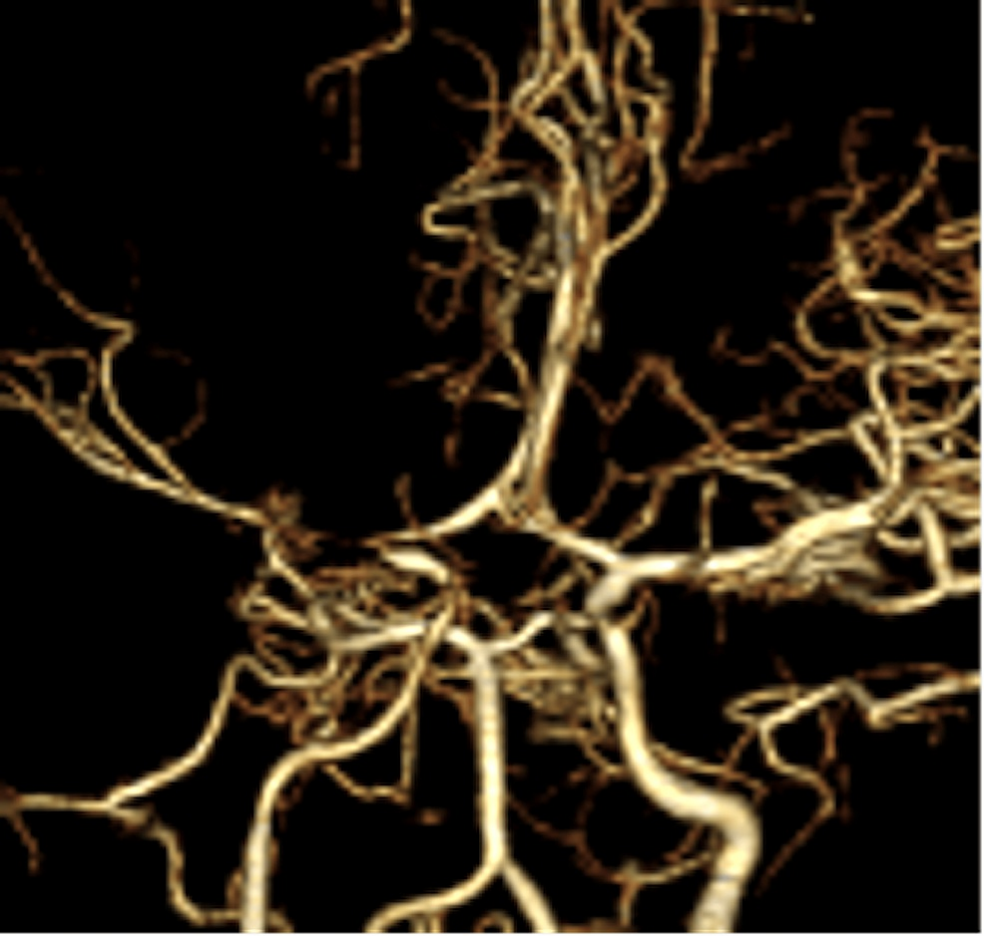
A CT angiography scan on the ninth day showed severe MCA stenosis, suspicious for cerebral vasospasm. CT, computed tomography; MCA, middle cerebral artery
